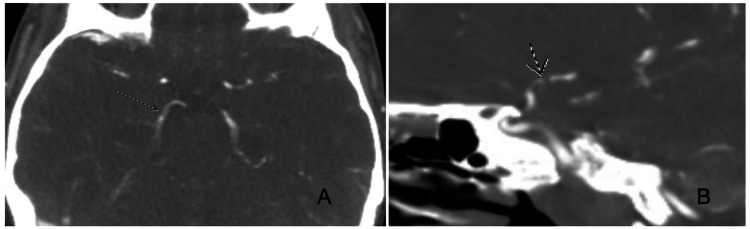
CT angiography (CTA) head demonstrating right posterior cerebral artery (PCA) multifocal stenoses throughout its course in the (A) axial section, including a moderate to high-grade stenosis within the proximal P1 segment, which is more appreciable in the (B) sagittal plane. These findings are consistent with reversible cerebral vasoconstriction syndrome (RCVS), a condition characterized by the transient narrowing of cerebral arteries. Stenosis is hypothesized to result from multiple pathophysiological mechanisms, including endothelial dysfunction, sensitivity to vasoactive substances, and sympathetic nervous system involvement.
